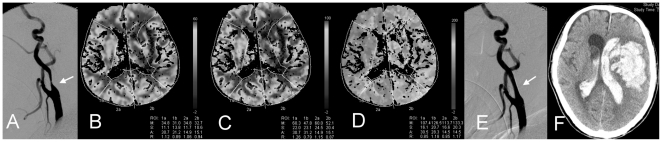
A 68-year-old man with a right hemispheric ischemic stroke. (A) Pre-stenting digital subtraction angiography reveals a 76% stenosis of the left internal carotid artery. A perfusion computed tomography (CT) study demonstrates a relatively decreased regional cerebral blood volume (rCBV) (B), decreased regional cerebral blood flow (rCBF) (C), and increased time to peak (TTP) (D) in the left hemisphere. The stenting procedure shows a 25% residual stenosis of the carotid artery (E). The rainbow on the right display reveals a range of rCBV from −2 to 60 ml/100 gm; rCBF from −2 to 100 ml/100 gm/min and TTP from −2 to 200 deciseconds. The patient suffered a sudden loss of consciousness two hours after stenting, and follow-up brain CT reveals a massive hematoma of the left basal ganglia, left frontal and temporal lobes with rupture into the ventricles (F).
